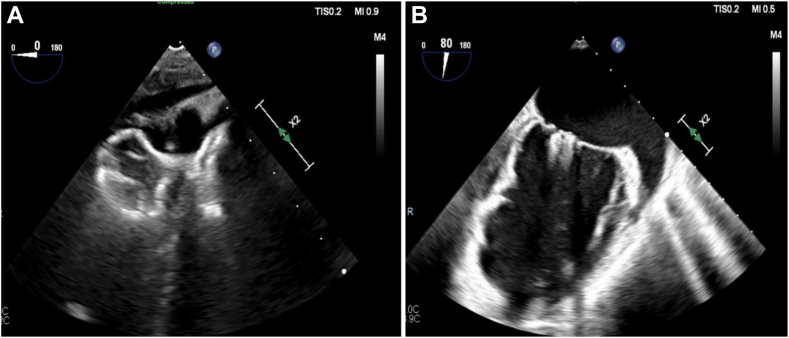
Transesophageal echocardiogram (after repair). (A) Amplatzer occluder device occluding ventricular septal rupture. (B) Two MitraClips for mitral valve repair.
